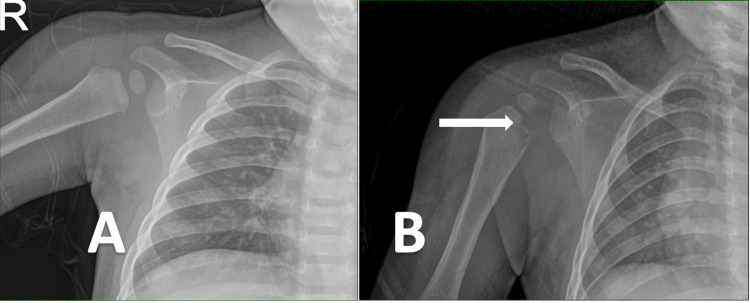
Plain anteroposterior radiograph of the right shoulder at first presentation (A) and second presentation (B). In radiograph B, there was a lytic lesion over the proximal humerus indicated by the arrow.
